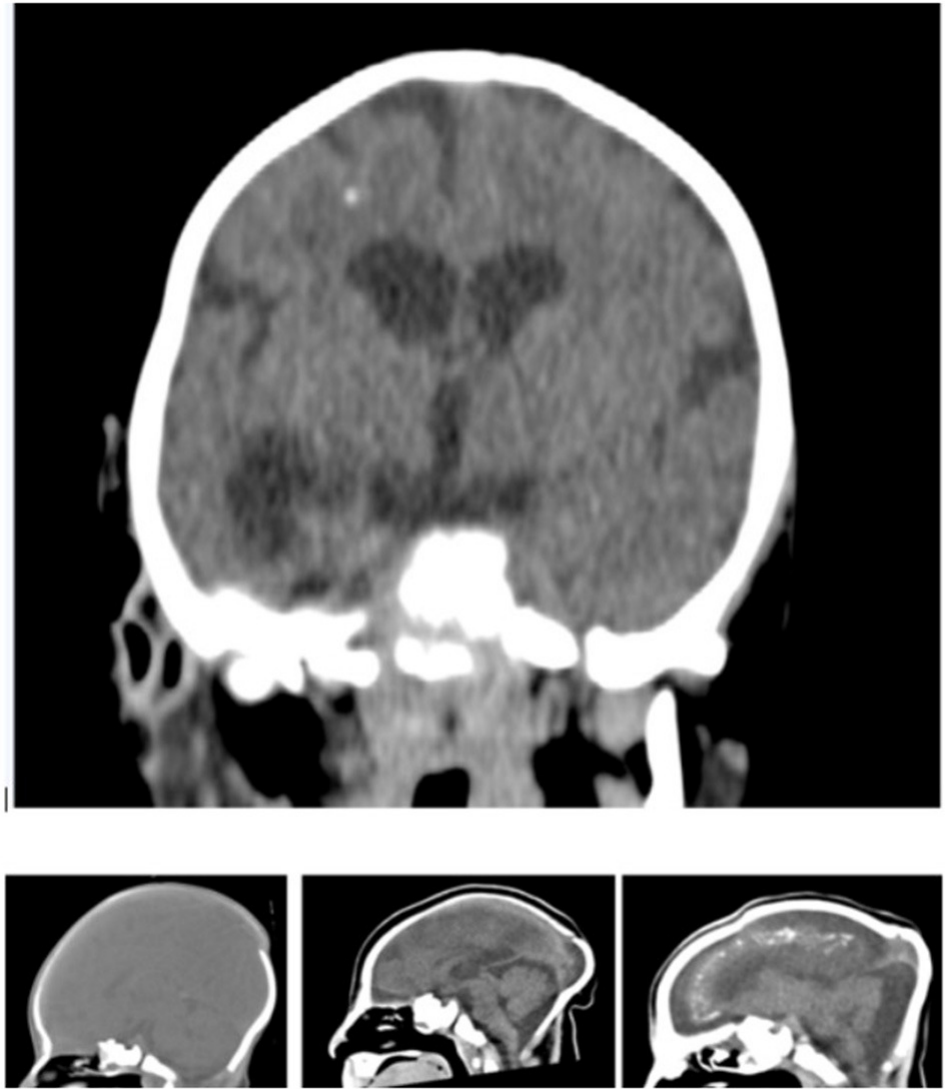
Coronal view of a non-contrast CT of an infant infected by Zika virus showing supratentorial volume loss and ventriculomegaly, pachygyria and foci of white matter calcification and sagittal images showing the normal and the moderate and severe parenchymal volume loss.
